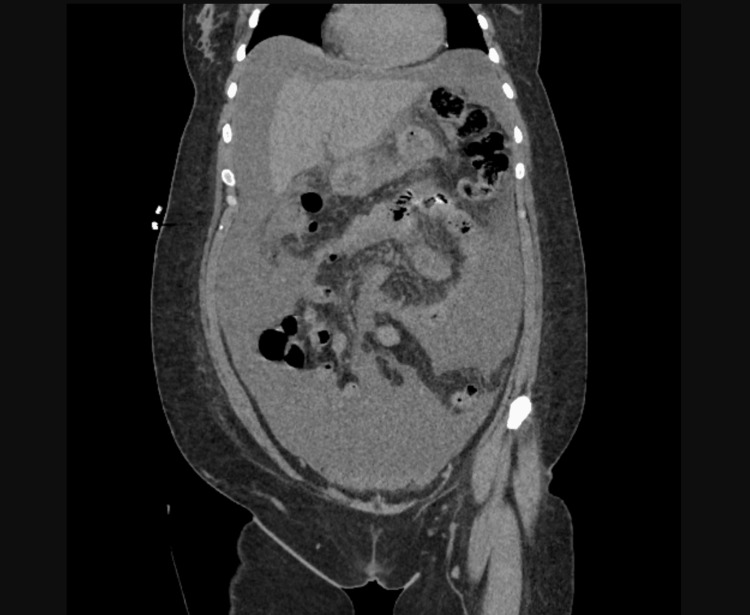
CT abdomen pelvis showing complex intra-peritoneal ascites
